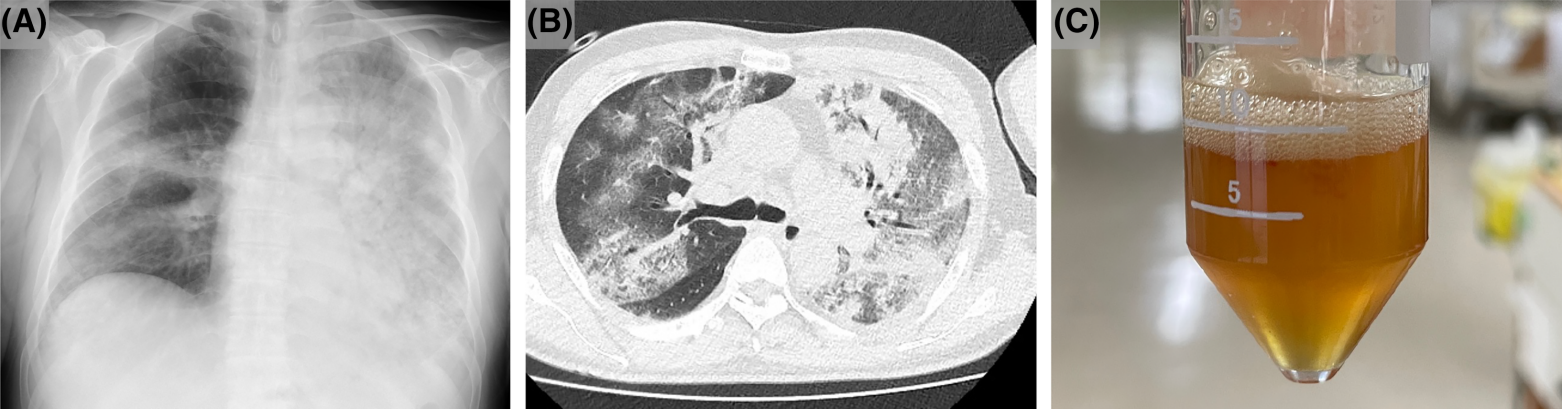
(A) Chest x‐ray shows extensive infiltrative shadows in the bilateral lungs. (B) Chest computed tomography shows extensive ground‐glass opacity and consolidation. (C) Bright orange, very watery airway secretions aspirated using a suction tube.
